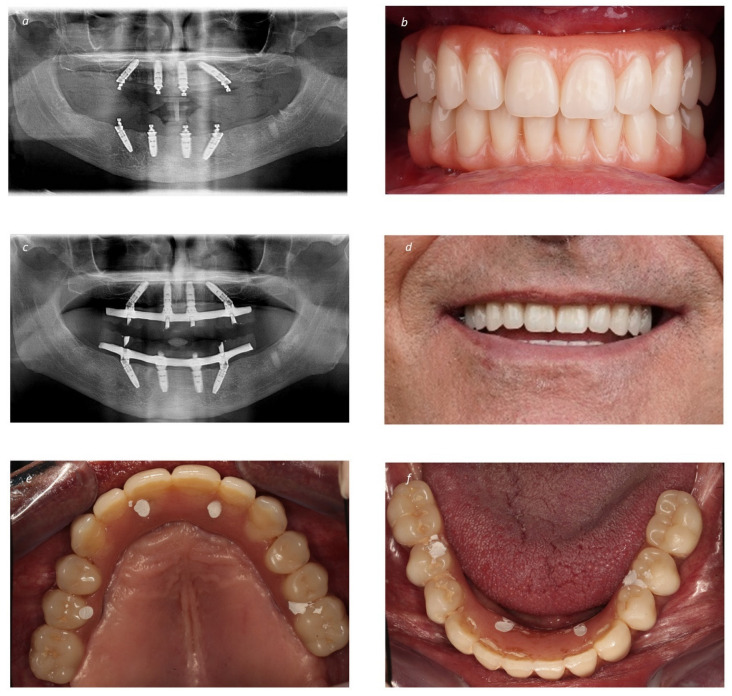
Prosthetic restoration of the bimaxillary full-arch rehabilitation: (a) Post-operative orthopantomography immediately after surgery; (b) Intraoral frontal photograph with the immediate provisional prostheses; (c) Final orthopantomography at 2 years of follow-up with the definitive prostheses (Maló Clinic Acrylic Bridge—titanium infrastructure, acrylic artificial gingiva and acrylic crowns); (d) Patient smiling with the definitive prostheses in place at 2 years of follow-up; (e) Intraoral occlusal view of the maxillary definitive prostheses at 2 years of follow-up; (f) Intraoral occlusal view of the mandibular definitive prostheses at 2 years of follow-up.
